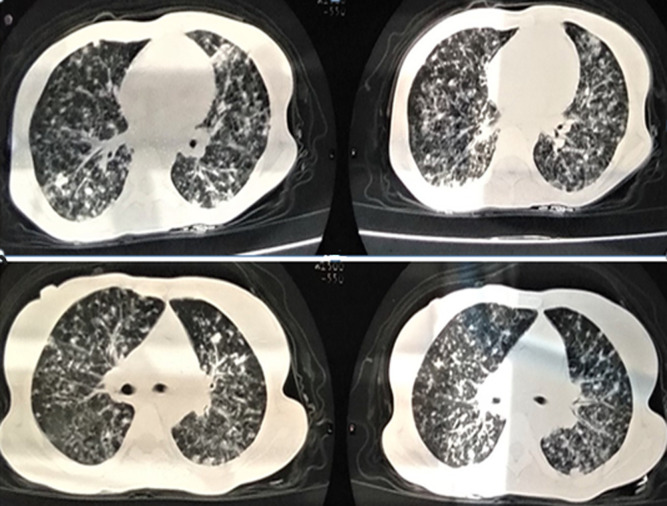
a thoracic computed tomography without injection of contrast product showing multiple micronodules, very likely in relation to a miliary tuberculosis, without typical signs of COVID-19
